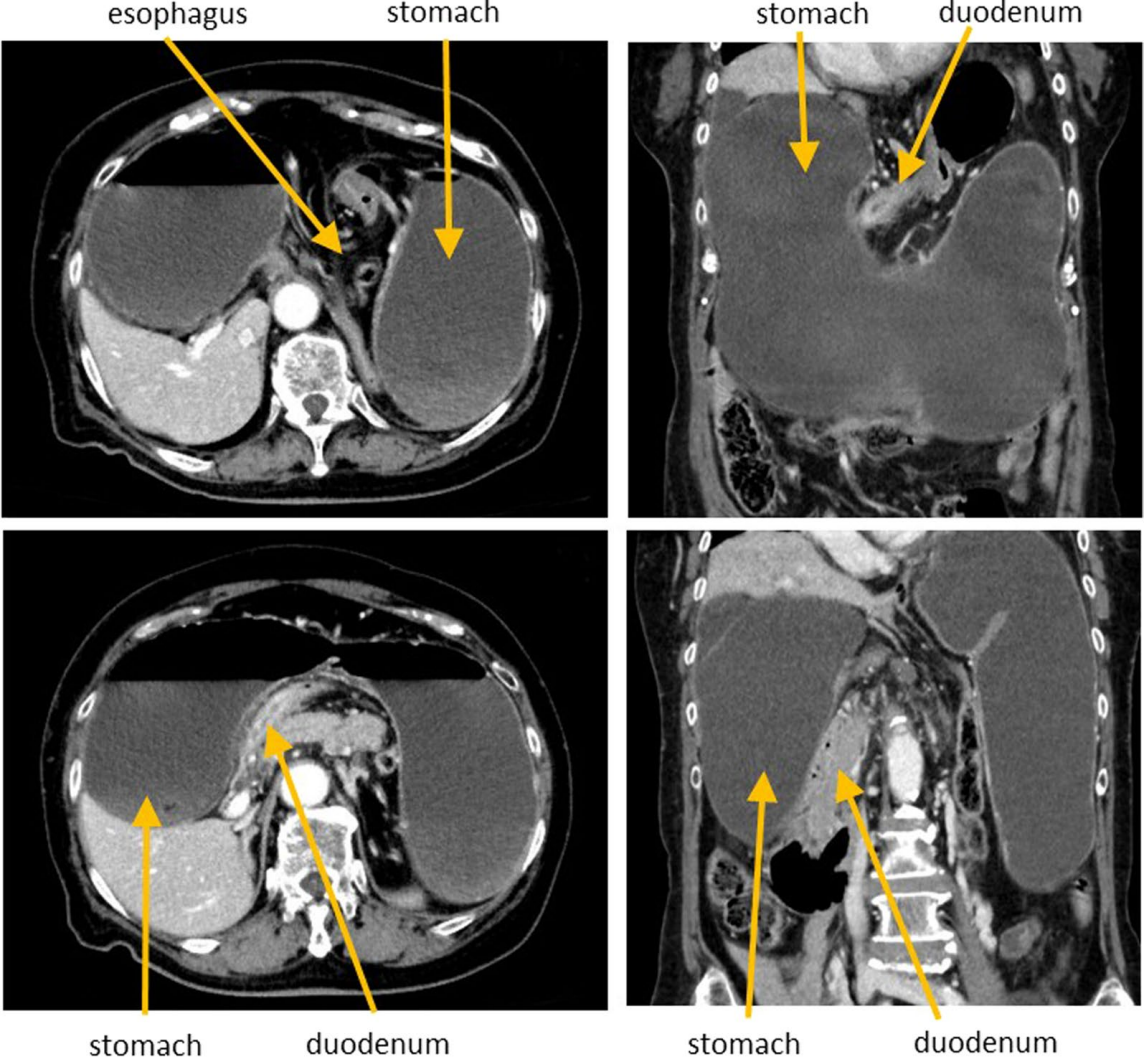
Abdominal computed tomography showed mesentero-axial gastric volvulus (MAGV) with markedly dilated stomach and stenosis of the duodenum
